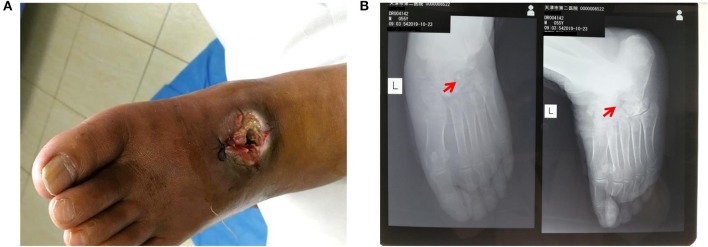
Post-operative detection showed successful debridement and ebonation. (A) Alleviated swelling presented on the surface of left-foot dorsum after debridement; (B) X-ray film view showed that the displaced intermediate cuneiform was removed. The red arrows indicate the position of removed intermediate cuneiform.
